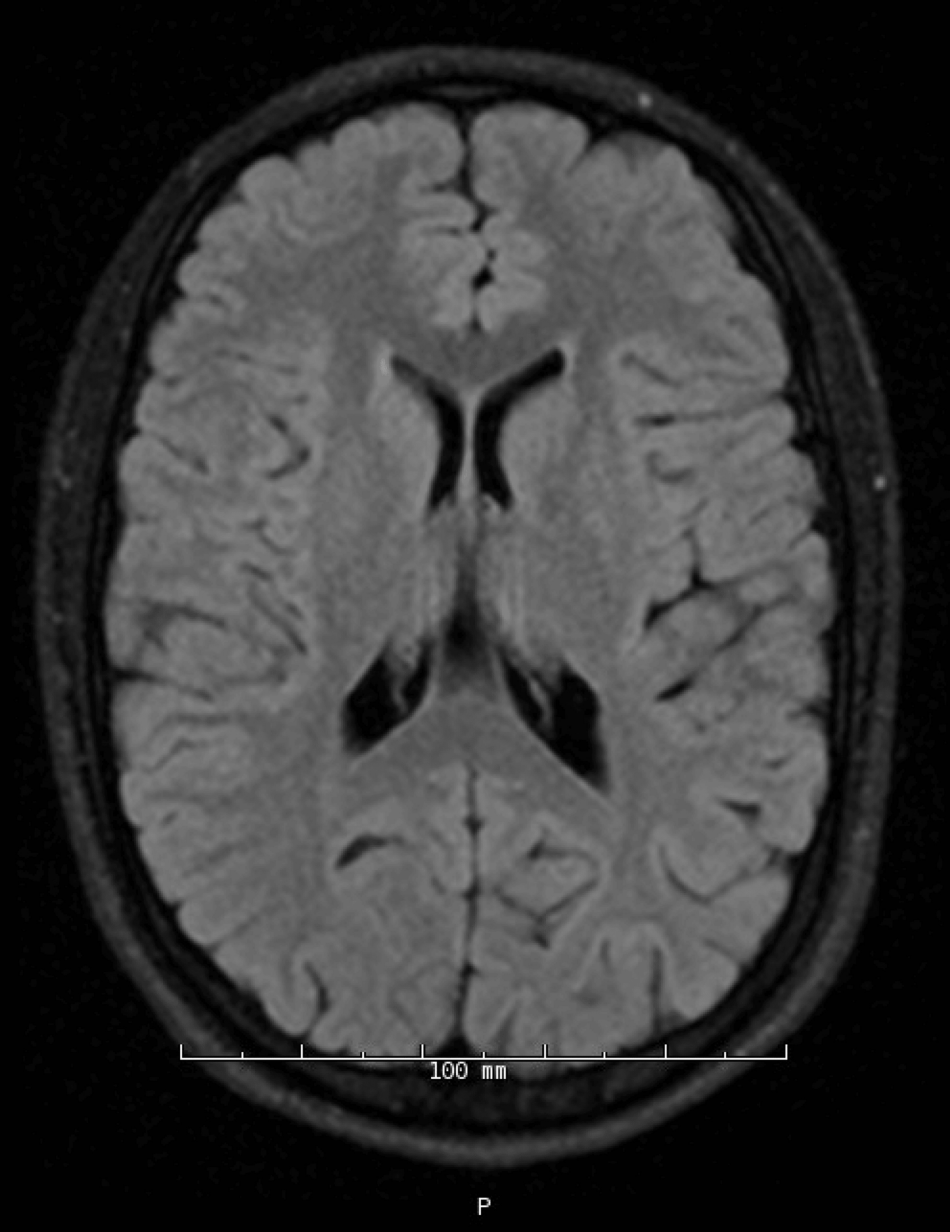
T2/FLAIR MRI brain without IV contrast
